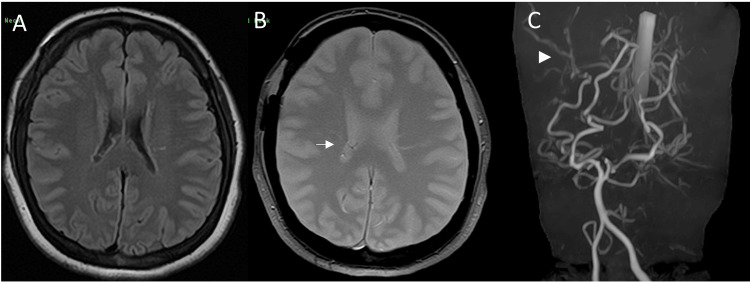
MRI scan five months after the aneurysm confirmation. A: The flow void signal with edema around the right lateral ventricle had disappeared. B: The T2 star image also showed no hemosiderin deposition. C: The peripheral aneurysm of the right lateral posterior choroidal artery had disappeared.
